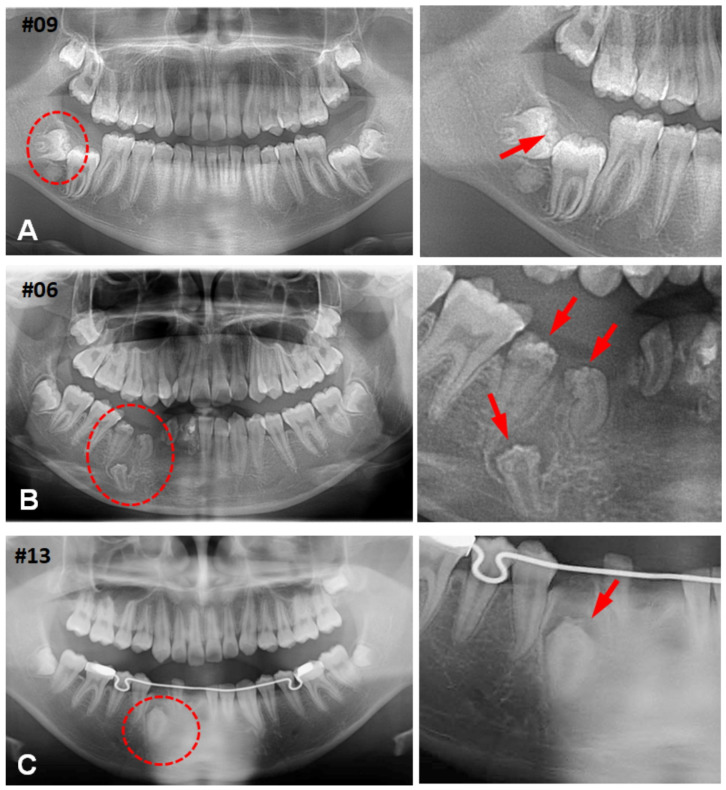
Representative dental panoramic radiographic images and enlarged pictures of dotted circles: a hyperplastic dental follicle (arrow) in case 9 affecting the impacted mandibular third molar (A); multiple calcifying hyperplastic dental follicles (arrows) in case 6 (B); and a solitary hyperplastic dental follicle (arrow) in case 13 (C).
